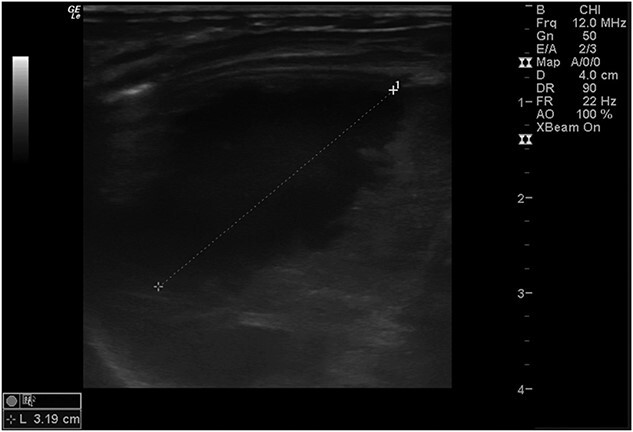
Abdominal ultrasound conducted 1 week post-operatively showed a possible splenic hematoma, which was self-limiting.
